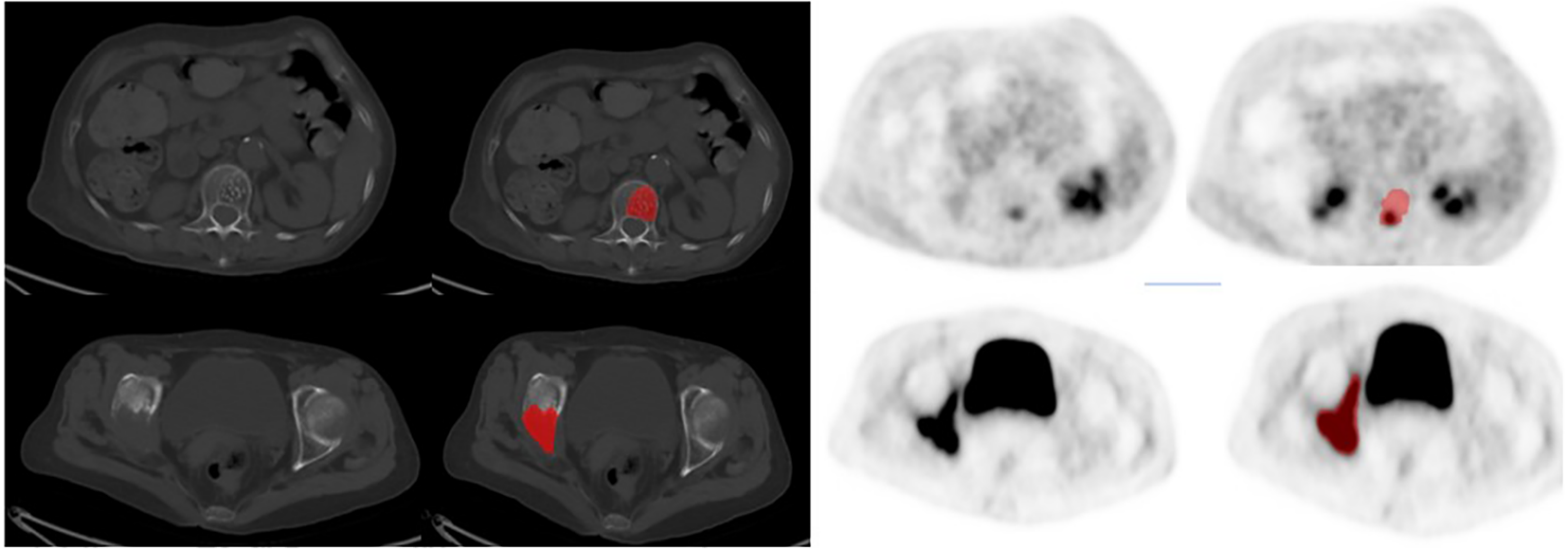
The typical imagings of two patients:The first row of images showed a 67-year-old woman, 6 months after right breast cancer surgery, CT showed the first lumbar had low density foci, and accompanied with multiple punctate high-density focals, PET images of this lesion local metabolism slightly increased, and finally diagnosed as benign hemangioma after 6 months of clinical follow-up. The second row of images showed a 72-year-old woman, 4 years after left breast cancer surgery, CT showed right sciatic and right iliac were all destructed, PET images of these lesions' metabolism were obviously increased, and finally diagnosed as bone metastasis with puncture pathology.
